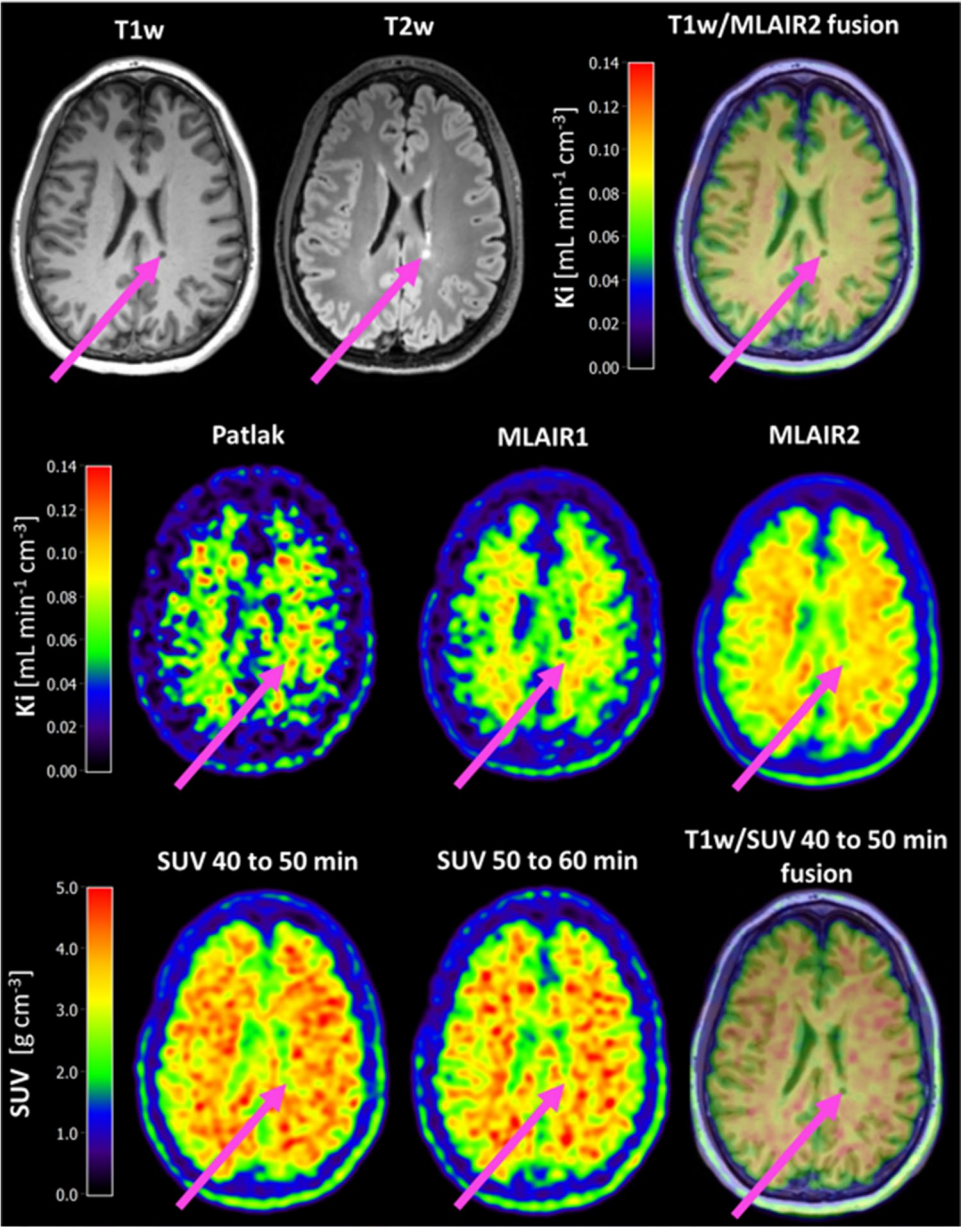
[^11^C]MeDAS PET images. The pink arrow indicates a MS lesion, which was hypo-intense on T1w MRI and hyper-intense on T2w-MRI. Decreased [^11^C]MeDAS uptake can be observed in MLAIR2, SUV 40–50 min, and SUV 50–60 min images, whereas the K_i_ images of Patlak and MLAIR1 are too noisy to clearly differentiate differences in myelin density. For the presentation purpose, the PET images were smoothed with a 4-mm Gaussian filter.
